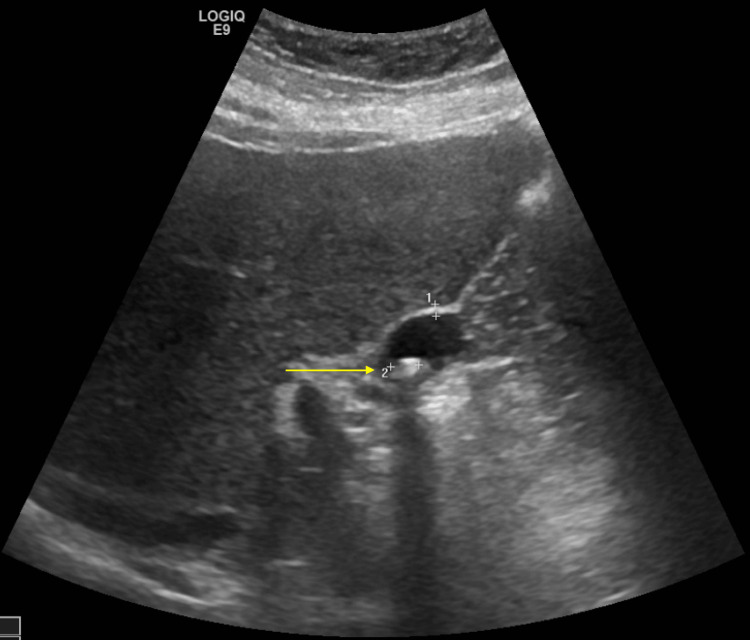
Right upper quadrant ultrasound showing a gallbladder remnant with wall thickness of 1 mm and the presence of an approximately 2 mm gallstone (yellow arrow). Multiple shadowing stones are noted. No pericholecystic fluid is seen. Sonographic Murphy's sign is negative.
